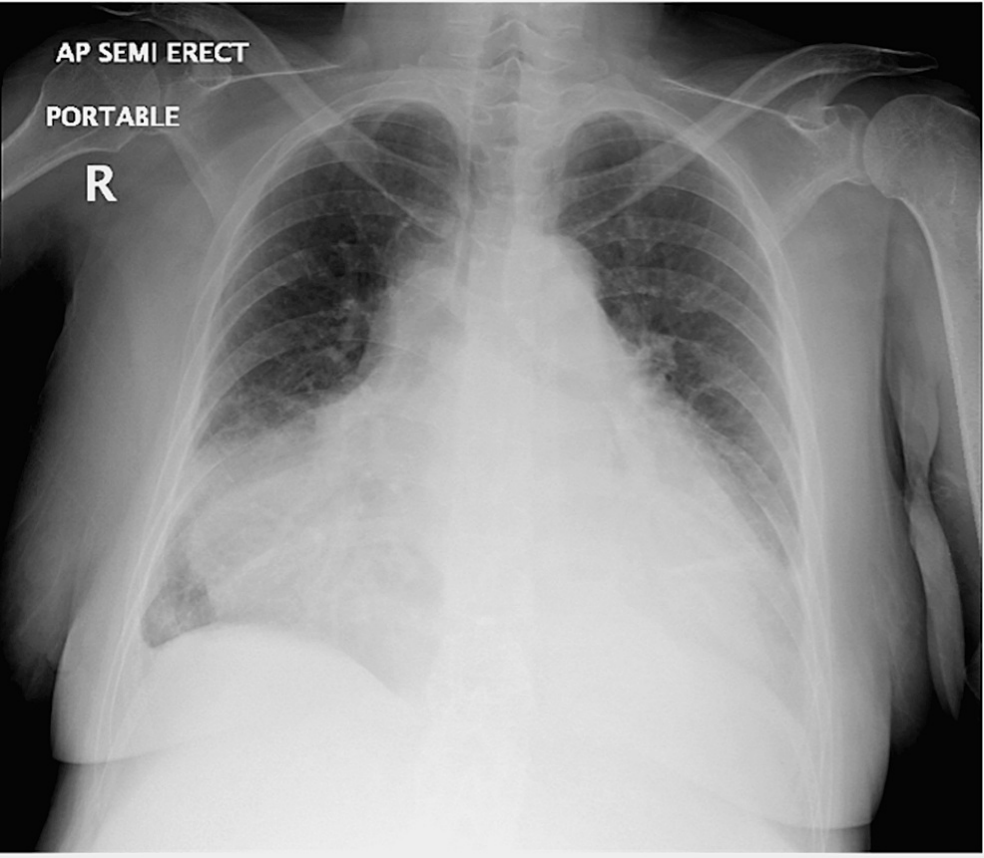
Chest X-ray. Enlarged cardiac silhouette corresponding to a “water bottle sign”
